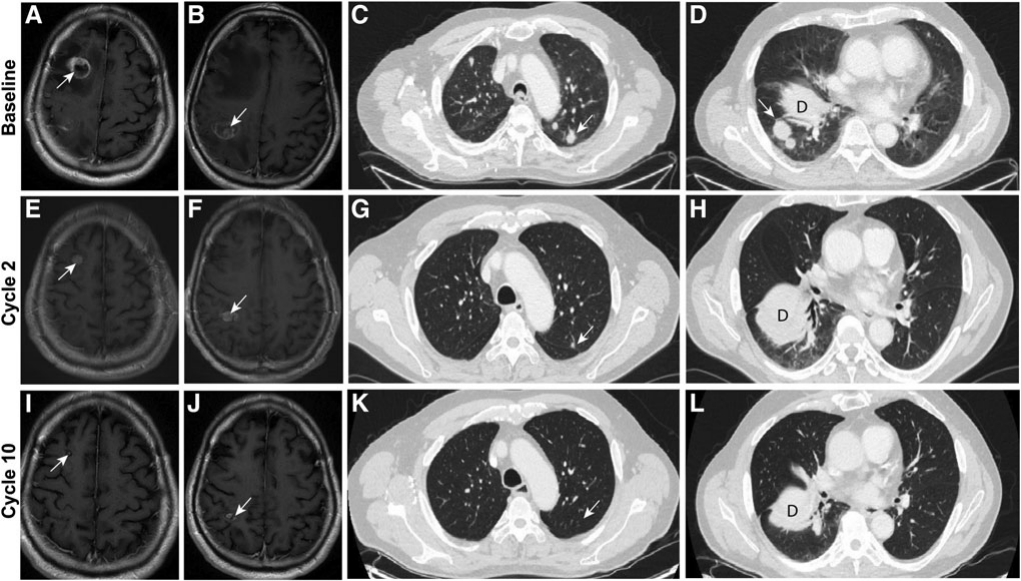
Magnetic resonance imaging of the patient's brain at baseline (A, B), at the end of cycle 2 (E, F) and cycle 10 (I, J) of LOXO-292 therapy showing progressive resolution of metastatic lesions. Computed tomographic images of chest lesions before (C, D) and at the indicated times after initiation of LOXO-292 (G, H, K, L). A radiological PR by RECIST Version 1.1 was achieved by the end of cycle 2 that deepened with continued treatment. PR, partial response.
